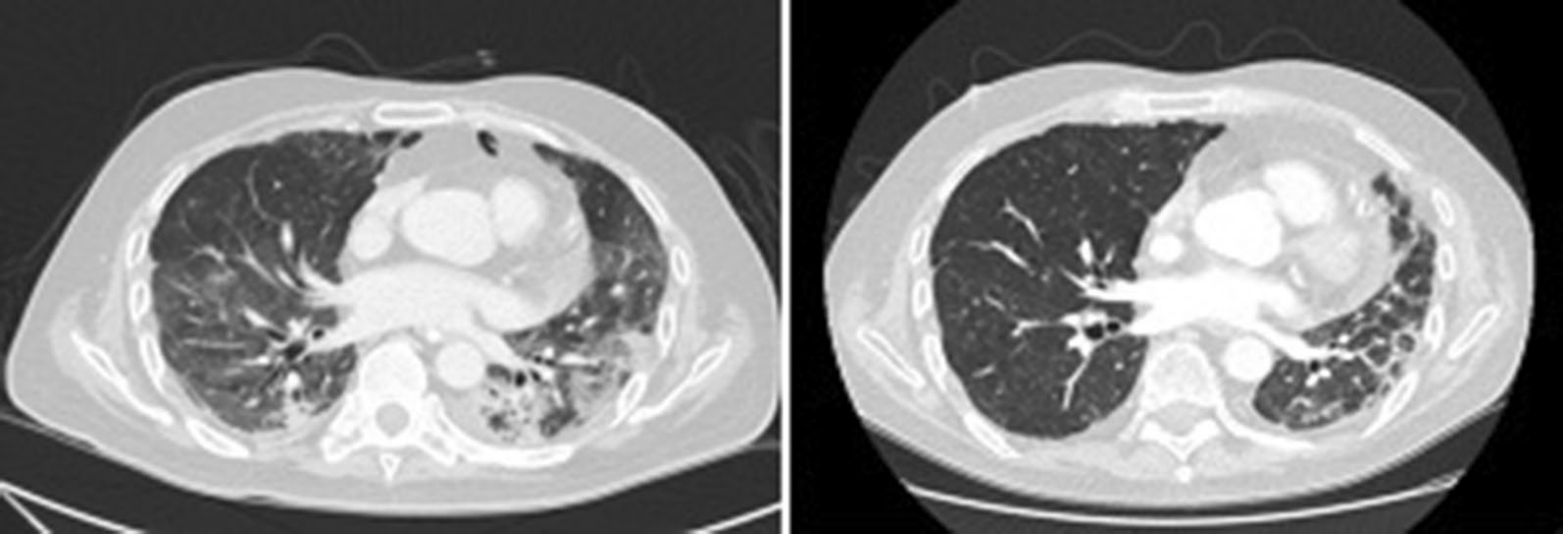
Chest CT scans. Left panel shows an interstitial infiltrate previous to ruxolitinib. Right panel shows 1-month chest CT scan follow-up with nearly complete resolution of the pulmonary infiltrates
